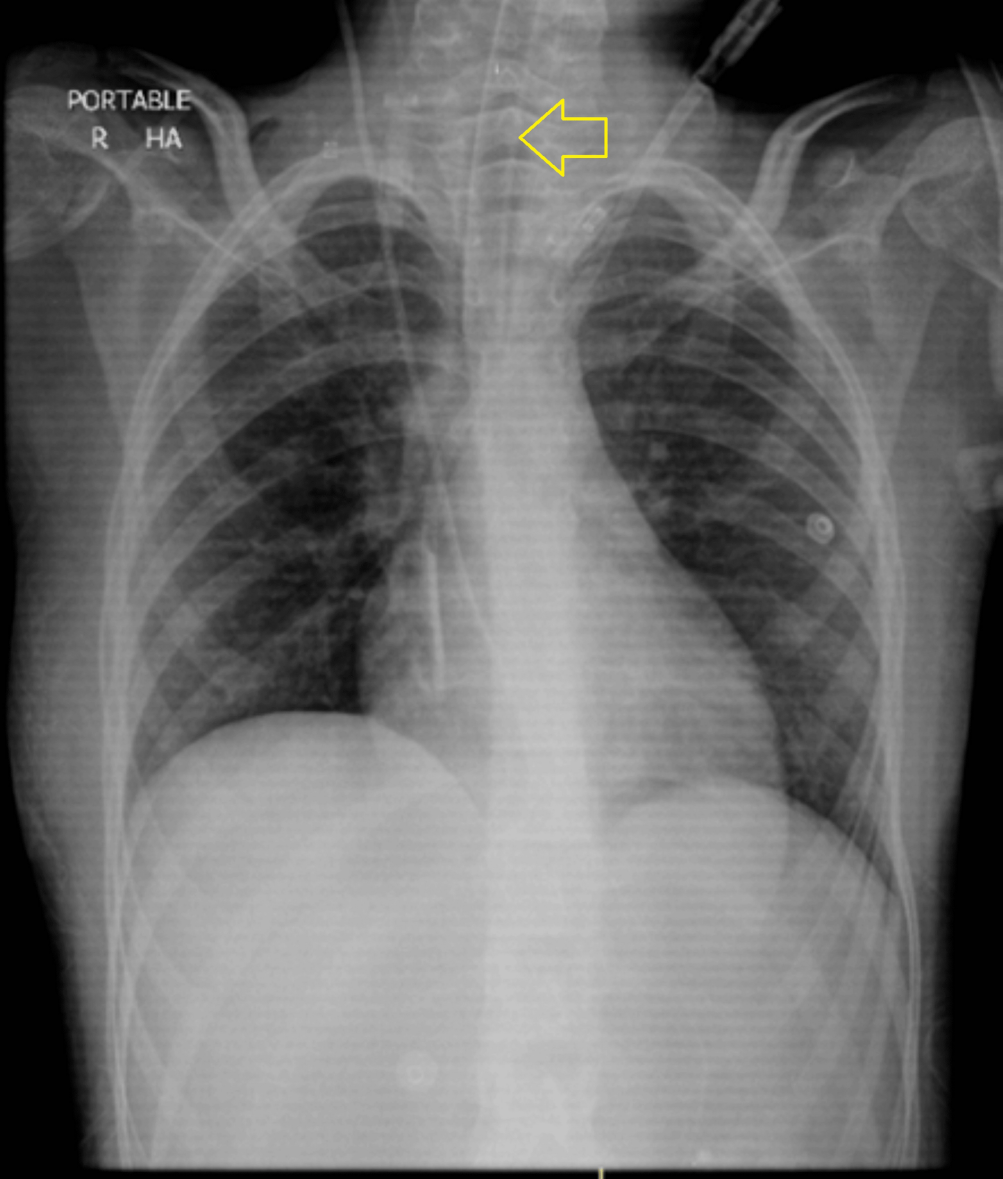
Chest X-ray post-chest tube removal with the endotracheal tube in place (yellow arrow)
